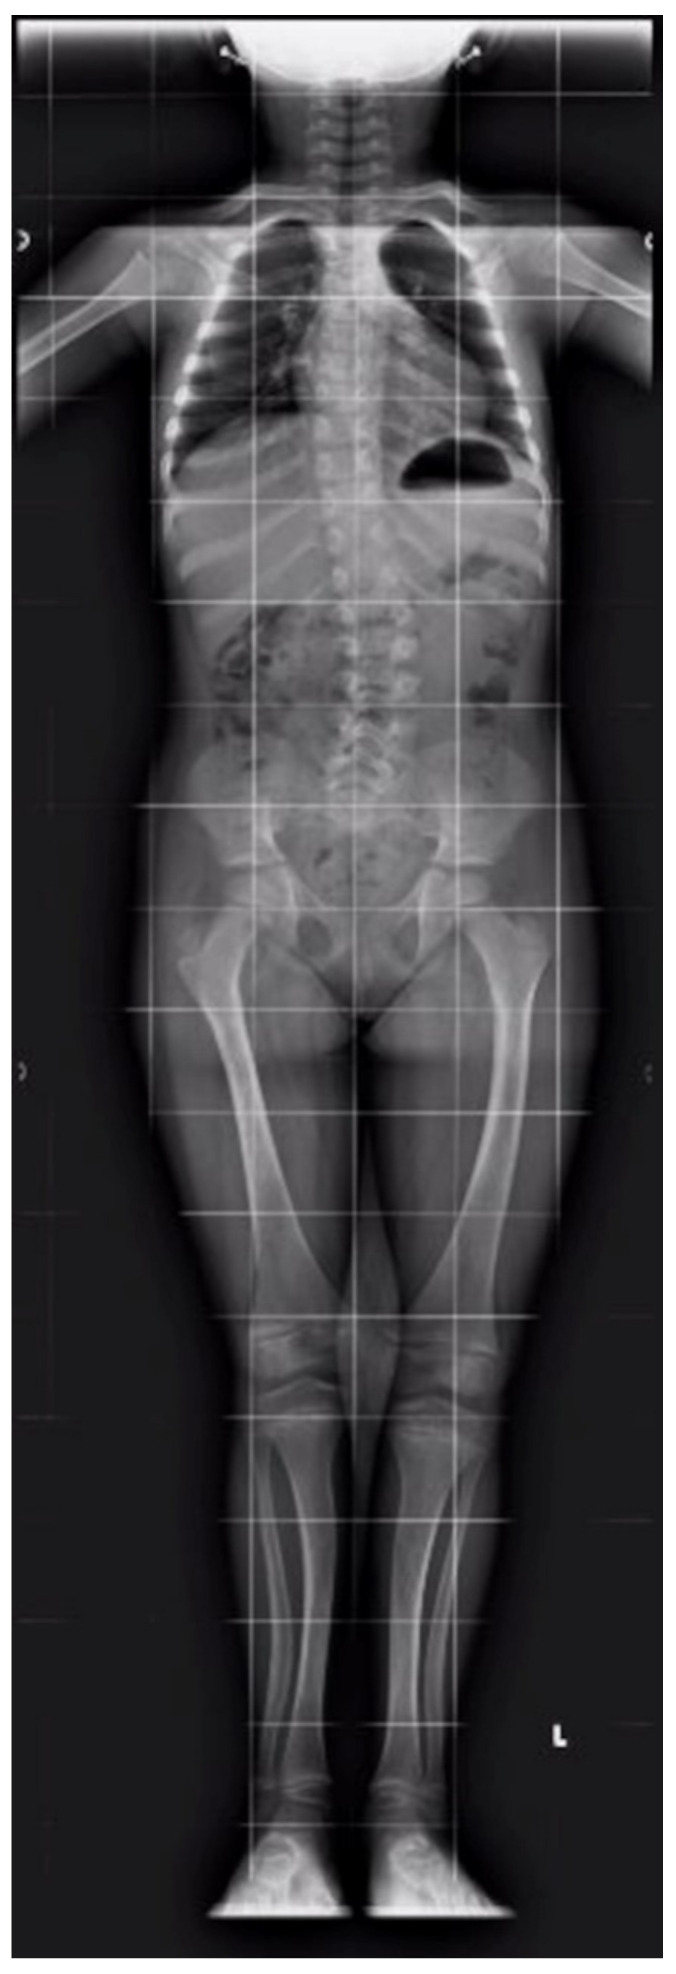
X-ray at the end of therapy.
